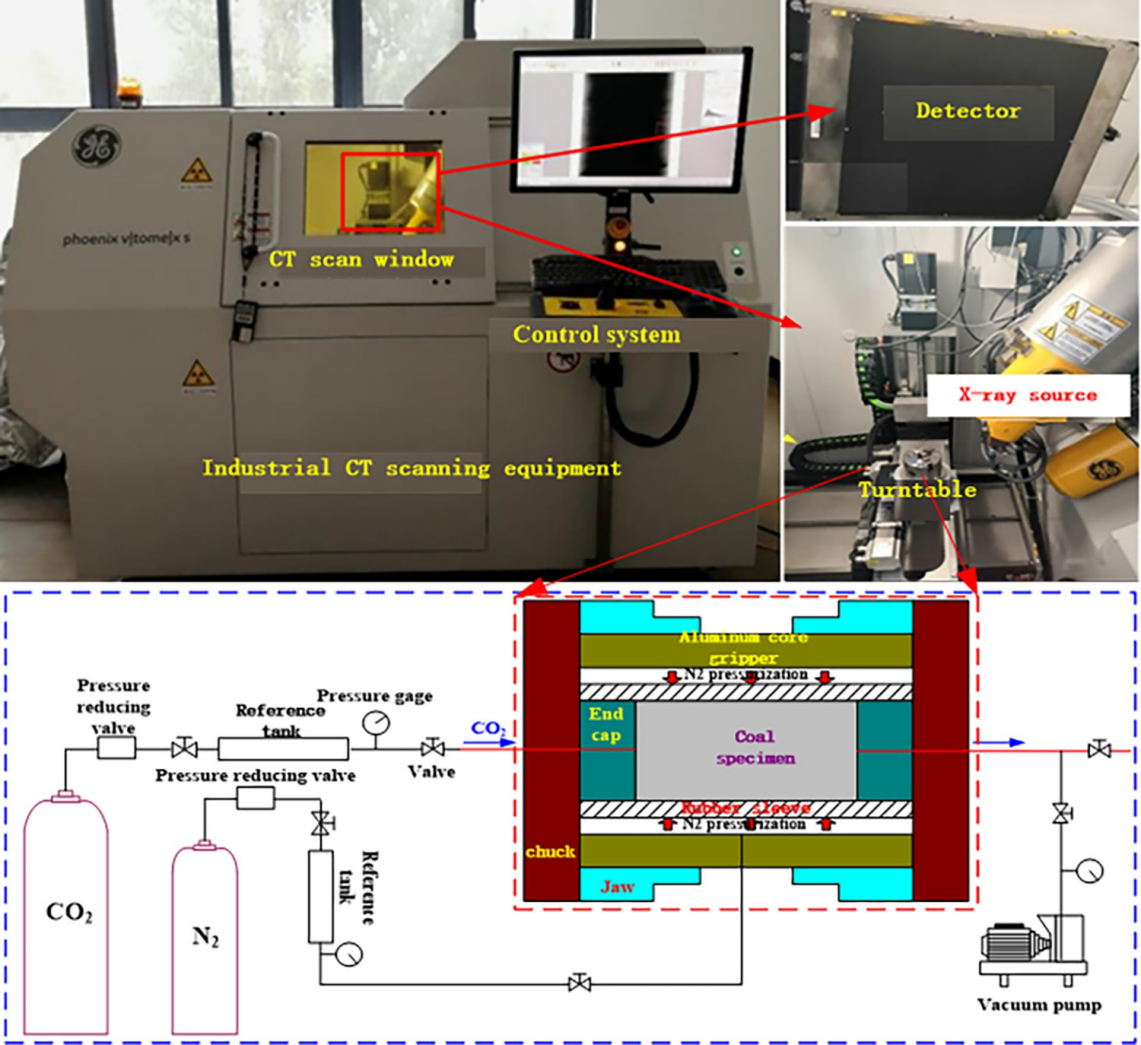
High precision CT scanning instrument and coal sample gas injection system.
